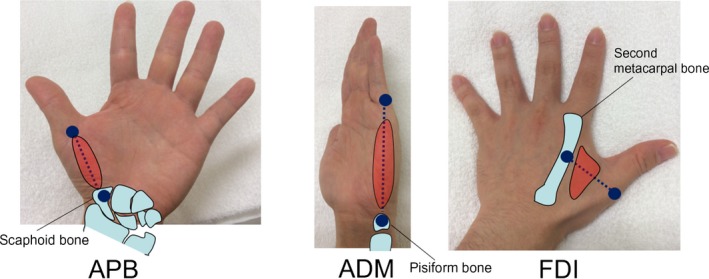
MUS recording sites for APB, ADM, and FDI muscles. The probe was placed along the line connecting the midpoint of the volar aspect of the first metacarpophalangeal joint and the volar prominence of the scaphoid bone for APB, along the line connecting the midpoint of the fifth metacarpophalangeal joint and pisiform bone for ADM, and on the dorsal surface of the hand perpendicular to the axis of the second metacarpal bone at the level of the first metacarpophalangeal joint for FDI. MUS, muscle ultrasound; APB, abductor pollicis brevis; OP, opponens pollicis; ADM, abductor digiti minimi; FDI, first dorsal interosseous
